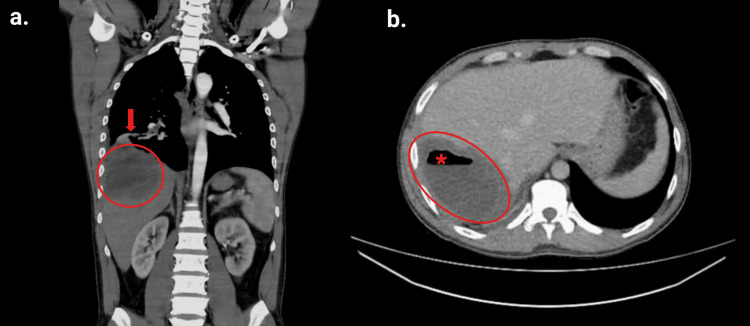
Contrast-enhanced abdominal and pelvic CT: (a) coronal view and (b) axial view. A multiloculated hypodense lesion with thick walls and an internal air-fluid level (red asterisk), consistent with a hepatic abscess (red circles), is identified in segments VII and VIII of the right hepatic lobe. The lesion measures 12.3 × 7.7 × 8.1 cm in the anteroposterior, transverse, and craniocaudal dimensions, respectively. Loss of the normal plane of separation between the hepatic parenchyma and the diaphragm is noted, with identification of a small posterior diaphragmatic defect (red arrow) measuring approximately 4.7 mm, associated with a cavitary lesion suggestive of a right basal pulmonary abscess (see Figure 2), raising suspicion of hepatopulmonary communication. CT: computed tomography
